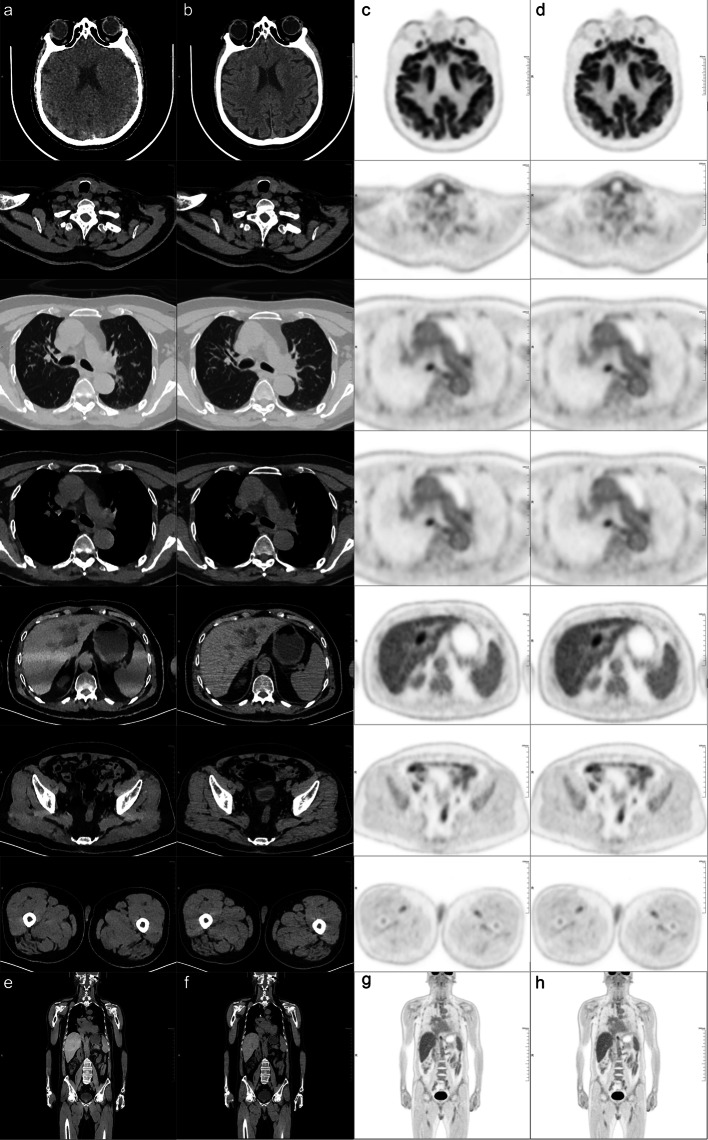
PET/CT images of a 66-year-old patient with intrahepatic cholangiocarcinoma. (a, b) column: transverse views of the CT images with ULDCT-AIIR and SDCT-HIR. c, d column: transverse views of the PET images with PET-AIIR and PET-HIR. e, f: coronal slice of the CT images with ULDCT-AIIR and SDCT-HIR. g, h: coronal slice of the PET images with ULDCT-AIIR and SDCT-HIR
